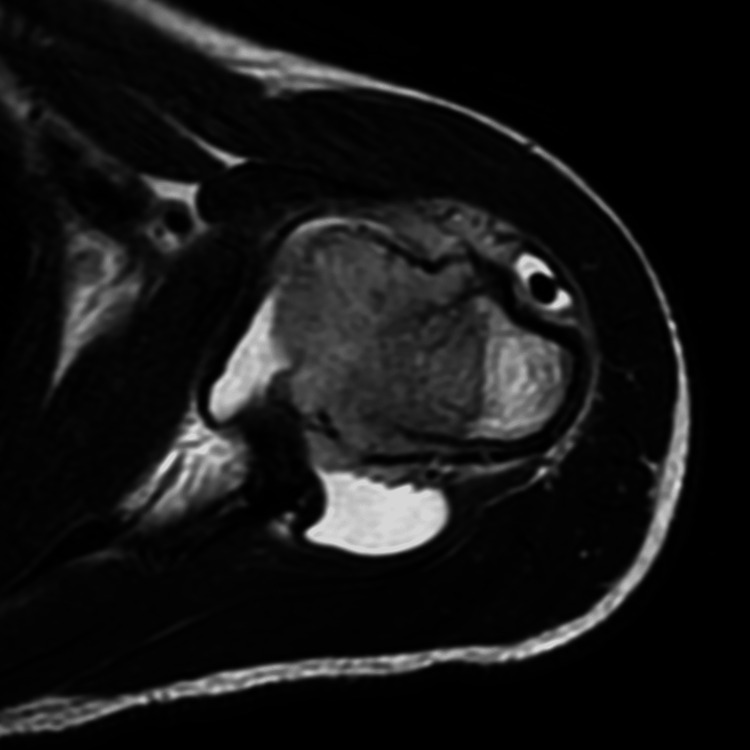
T2-weighted axial images at the level of humeral neck Extensive subperiosteal component of the mass is noted with breach of cortex as well as the overlying periosteum.
